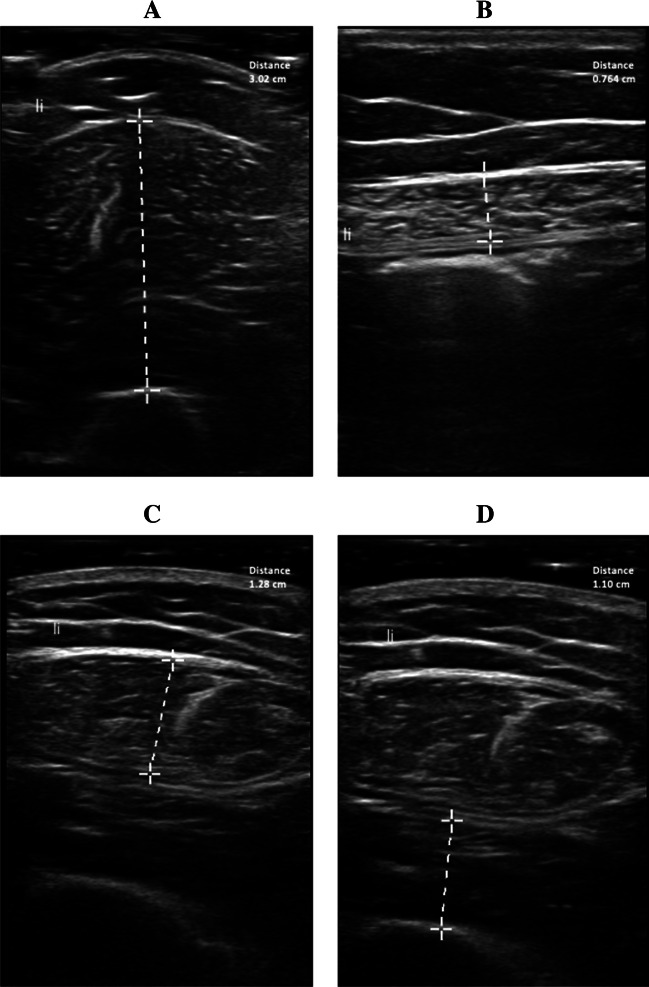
Representative images of the ultrasound muscle thickness measurements of the A m. biceps brachii, B m. rectus abdominis, C m. rectus femoris, and D m. vastus intermedius
